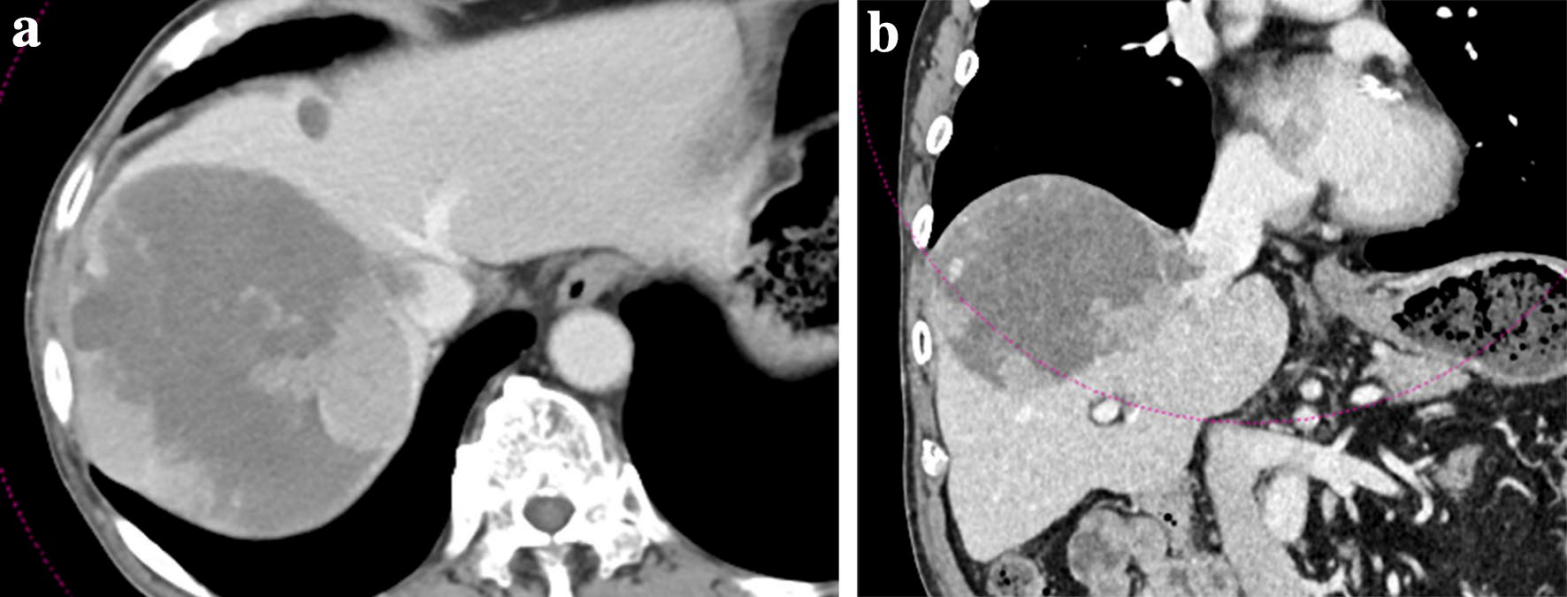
CT findings after 10 months of regorafenib treatment. Shown are the tumor characteristics from the a horizontal and b frontal plane. Though graded as stable disease, an 18.6% reduction in tumor size and shrinkage of the IVC-HVTT can be seen. *CT* computed tomography, *IVC-HVTT* hepatic vein tumor thrombosis protruding into the inferior vena cava
